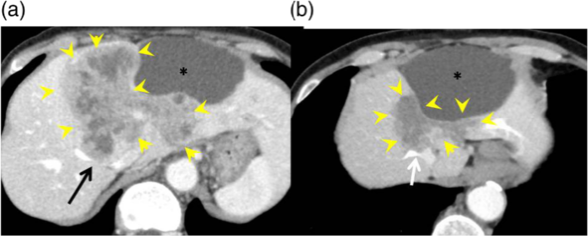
Multidetector-row computed tomography. a A large hepatic tumor occupying the left lobe and the right anterior sector of the liver (*yellow arrowheads*). The left and middle hepatic veins were occluded by invasion of the tumor, and the tumor also invaded the right hepatic vein (*black arrow*). b The liver tumor substantially decreased in size after chemotherapy (*yellow arrowheads*); however, it was still invading all of the major hepatic veins (*white arrow*). An asterisk indicates the liver cyst
